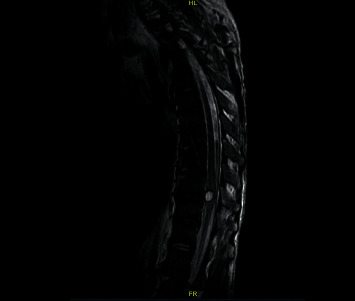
T2 MRI of the entire spine demonstrating a significant increase in the size of the previously noted lesion surrounded by a rim of a decreased gradient signal suggesting a hemorrhagic component and a likely picture of cord edema at T9-T10.
